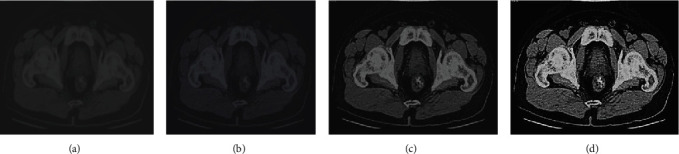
CT image of a patient with bladder cancer.
